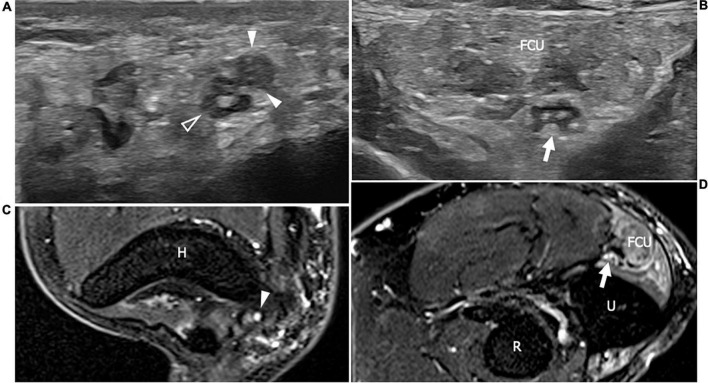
This figure shows two 18 MHz HRUS axial images (A,B) and two T2-weighted with fat suppression axial magnetic resonance (MR) images (C,D) in a 48 years old female patients with ANCA-associate vasculitis and a multifocal mononeuropathy of the ulnar nerve. (A) The axial view of the ulnar nerve (empty arrowhead) just cranial to the epitrochlear groove can be observed: the nerve is characterized by a single fascicle enlargement (white arrowheads) to which a focal T2-signal hyperintensity in the correlative MR image corresponds (white arrowhead in C). More distally, within the cubital tunnel, the ulnare nerve fascicles (white arrow) appear slightly enlarged and hypoechoic (B) with a s increase of T2-signal intensity in the matching axial MR image (white arrow in D). The flexor carpi ulnaris muscle (FCU) appears diffusely hyperechogenic in (B) as a consequence of denervation; in (D), the denervation process provokes an intramuscular edema seen to have increased the T2-signal within the muscular tissue. H, humerus; R, radius; U, ulna.
